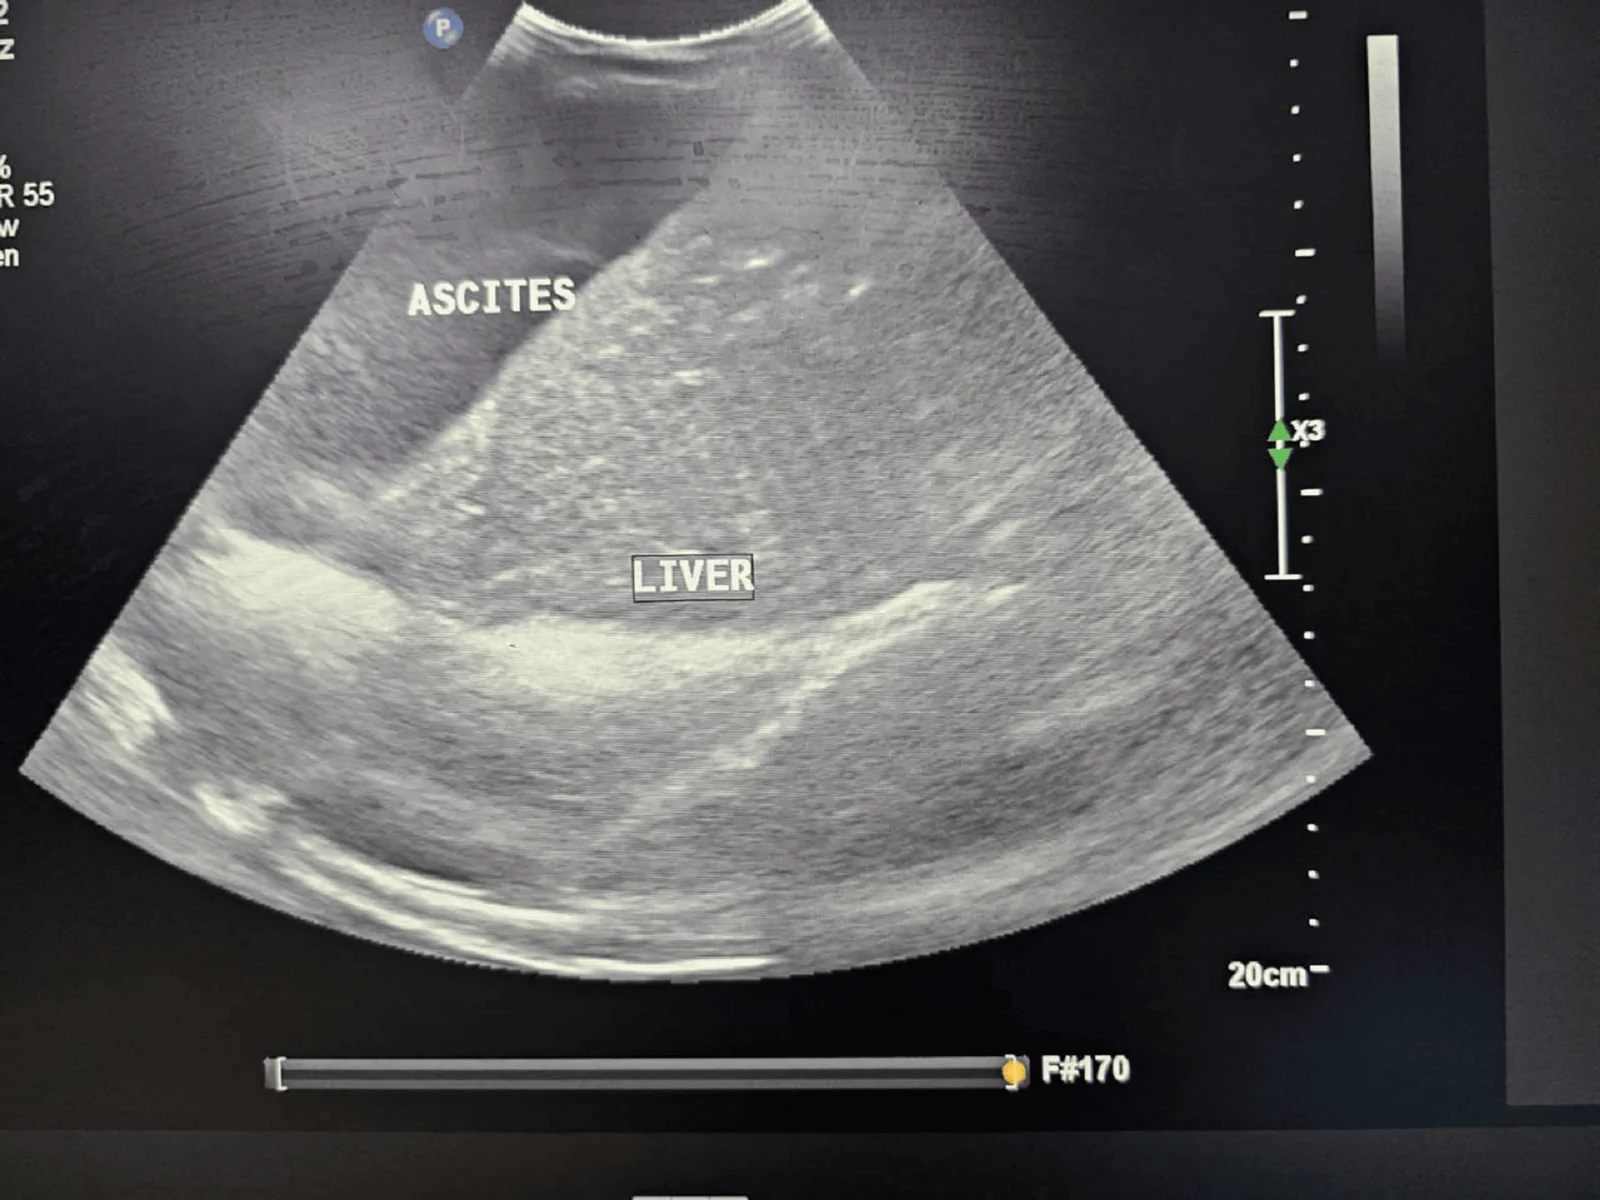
Abdominal ultrasonography showing moderate ascites. Abdominal ultrasonography demonstrating moderate ascites with preserved liver parenchyma, normal portal vein caliber, and normal renal architecture.
